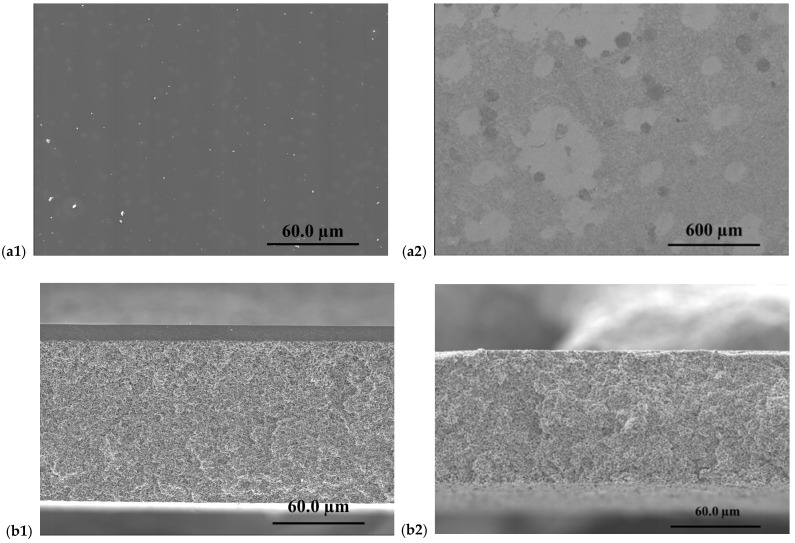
SEM images of surface and cross-section of CTA-based Durapore^®^–PIM (a1,b1), and PVC-based Durapore-PIM (a2,b2).
